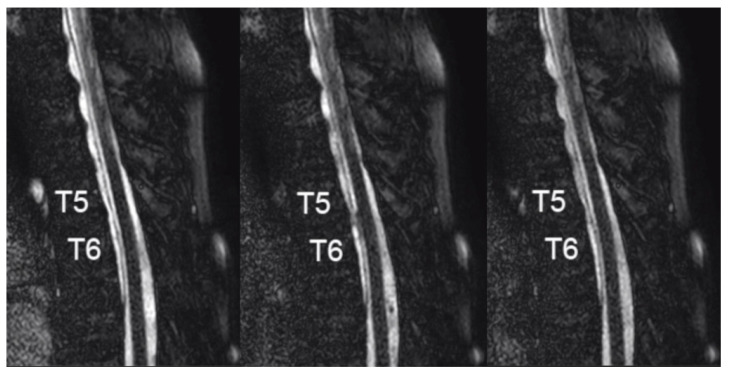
Images achieved by a dynamic improved motion-sensitized driven-equilibrium steady-state free precession (dynamic iMSDE SSFP) MRI sequence reveal an irregular flow of CSF through the dura at the T5–T6 level in the middle panel, confirming the position of the dural defect.
